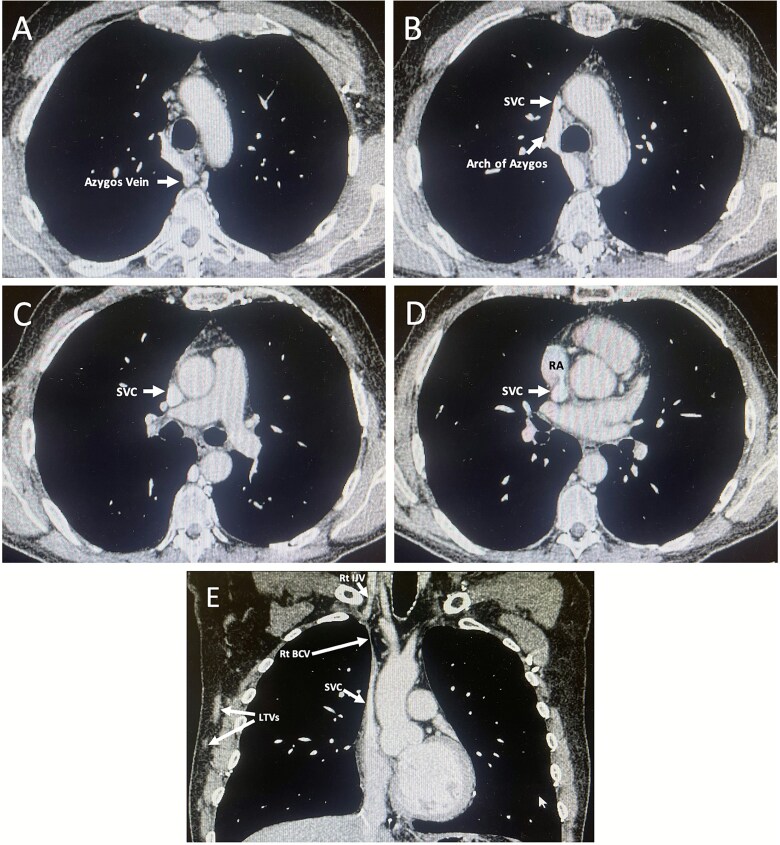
A-E: Contrast-enhanced CT of the thorax (venous phase). Transverse sections are shown in cranio–caudal order (A–D), with a coronal reconstruction (E).* (A) Axial image at the level of the aortic arch demonstrates a markedly dilated azygos vein draining into a prominent arch of azygos. The superior vena cava (SVC) is not visualised at this level, consistent with complete occlusion. The brachiocephalic veins—Which normally converge to form the SVC at this level—Are extremely difficult to appreciate, in keeping with non-opacification due to underlying occlusion. (B) Axial image immediately caudal to (A) again shows a prominent arch of azygos draining into a severely stenotic upper SVC. (C) Axial image at the level of the pulmonary trunk bifurcation demonstrating persistent SVC narrowing. (D) Axial image at the SVC–right atrial (RA) junction, where the SVC is patent. (E) Coronal reconstruction demonstrating a patent right internal jugular vein (IJV), complete occlusion of the right brachiocephalic vein (BCV), and non-opacification of the left IJV and left BCV consistent with chronic occlusion. Severe upper SVC stenosis is present. Prominent right-sided lateral thoracic veins (LTVs) are visible, reflecting collateral venous drainage of the right upper limb.
